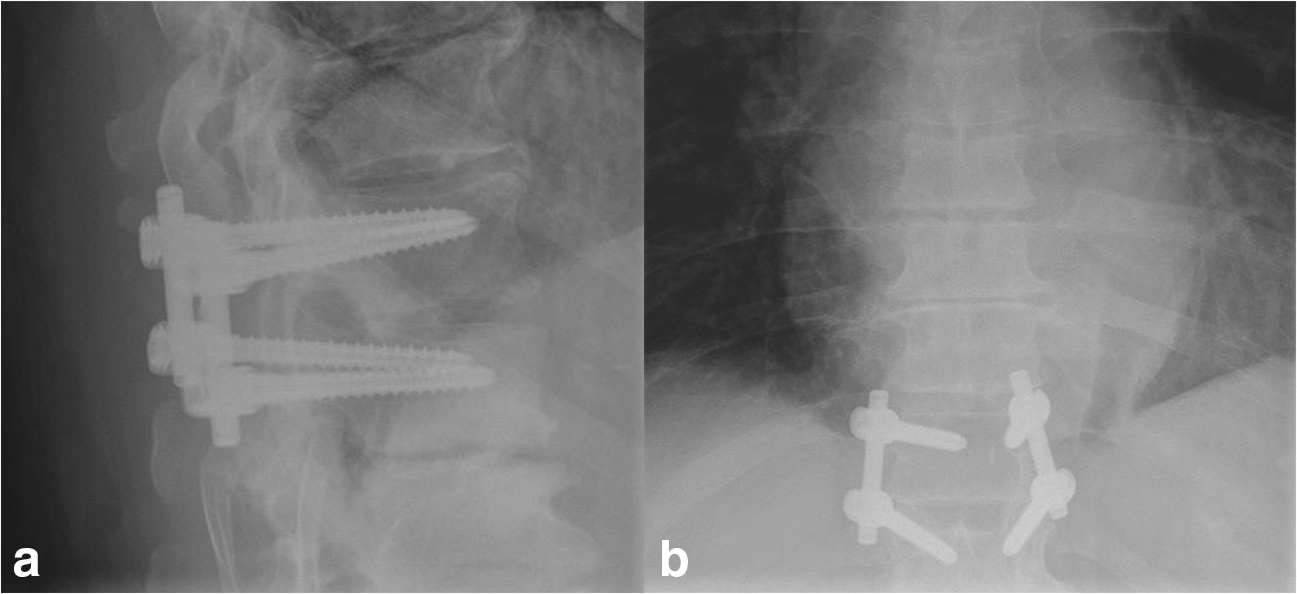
Postoperative X-ray in lateral (a) and anterior-posterior imaging (b)
